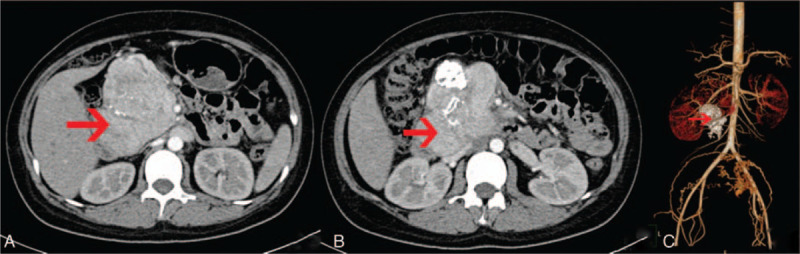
CT scan of the abdomen showing a highly vascularized retroperitoneal tumor measuring 9.3 × 7.3 × 11.8 cm with intratumor calcifications (A, B) and three-dimensional reconstruction CT of abdominal vessels and the tumor (C). No other tumor localization in the abdomen nor enlarged lymph nodes were detected.
